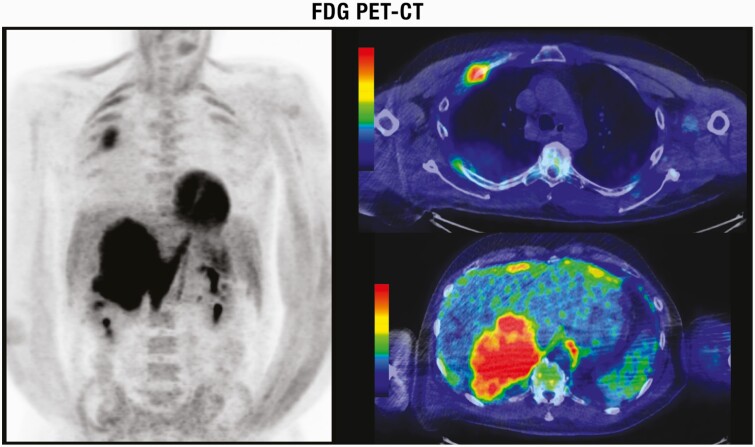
^18^F-fluorodeoxyglucose PET-CT. Coronal PET maximum intensity projection and axial fused PET-CT images in a patient with a locally advanced right adrenocortical carcinoma and an unsuspected tracer-avid right-sided rib metastasis.
